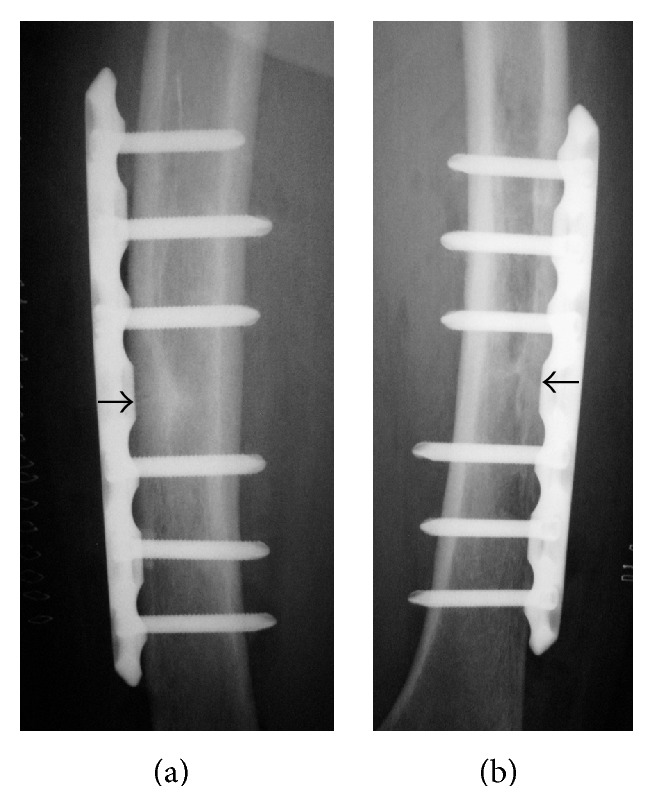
Anteroposterior radiographs of the bilateral femurs treated surgically using locking plates. Black arrows show original horizontal line.
